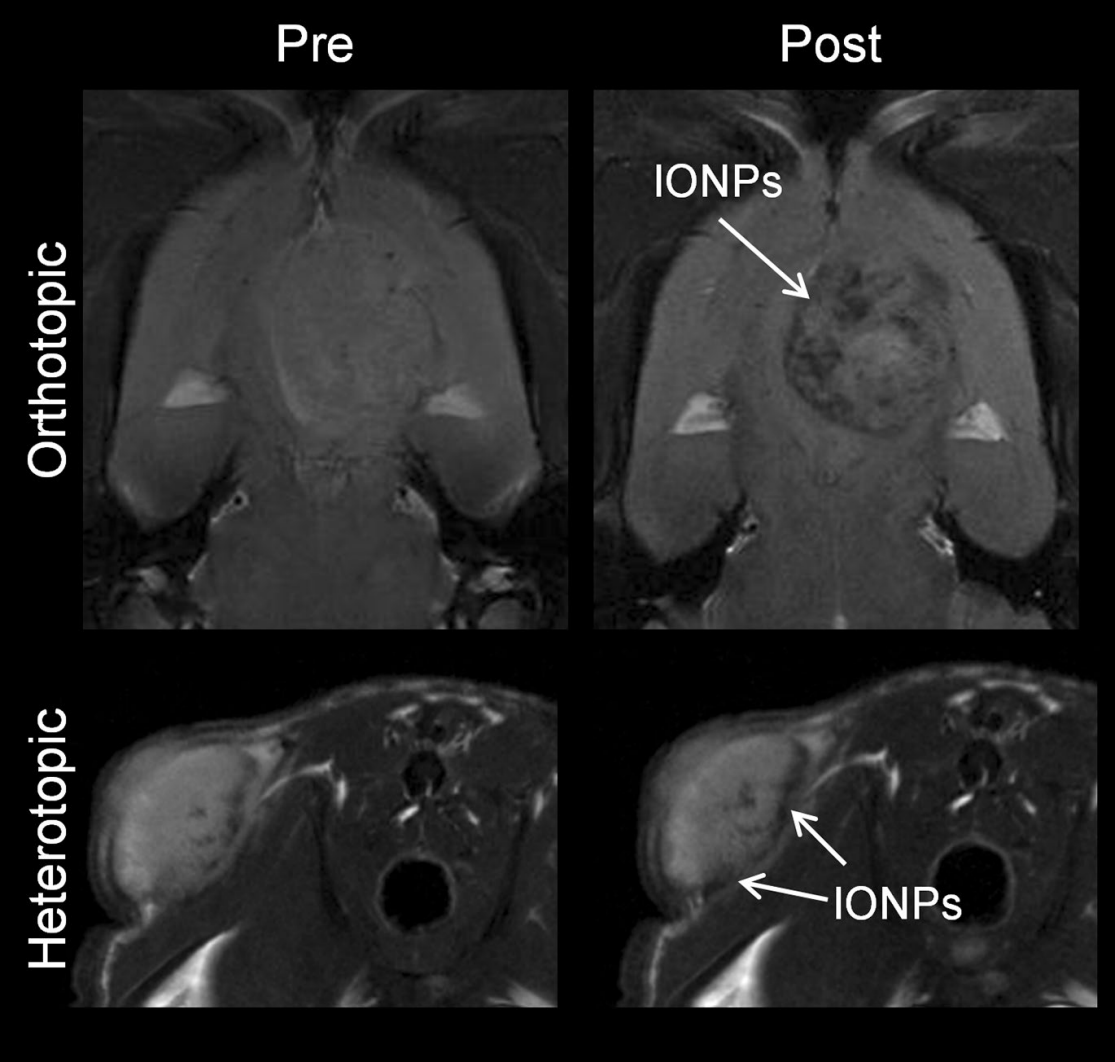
C6 brain tumor model implanted orthotopically (upper panels) and heterotopically (lower panels). Left) T_2_-weighted MR images before the injection of IONPs; right) T_2_-weighted MR images 1 h after the injection of IONPs
